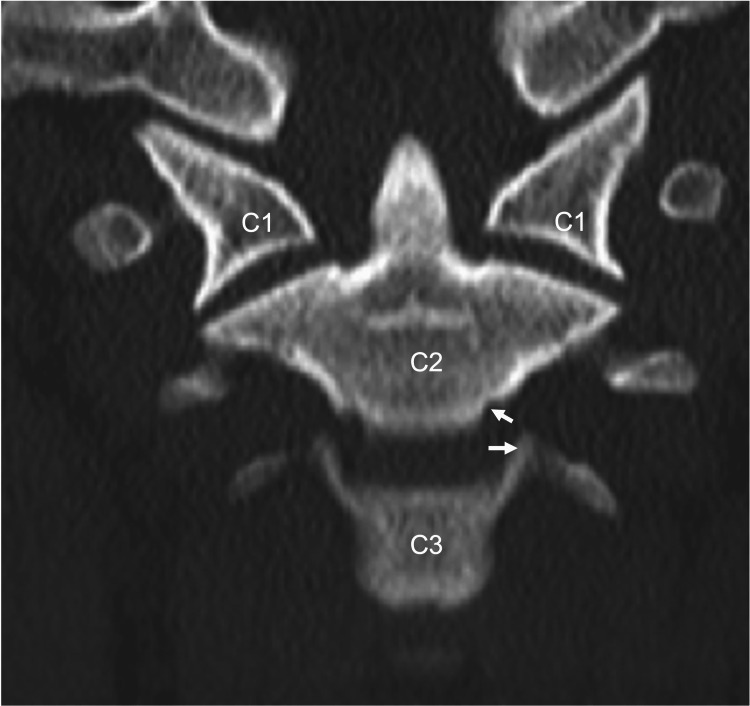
Coronal CT noting the énchancrure (upper arrow) of C2 and uncinate process (lower arrow) of the C3 vertebrae.
